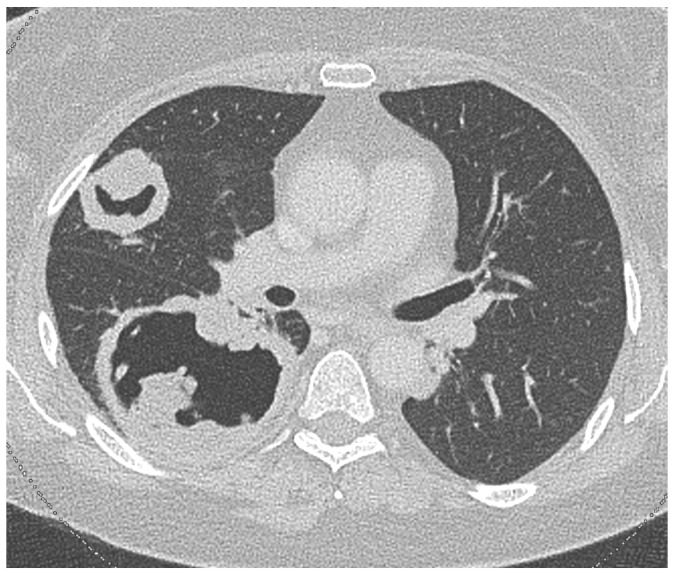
Chest CT: two cavitary lesions in the right upper and lower lobes, with the lower cavity containing a fungal mass with a thickened wall, consistent with aspergilloma. Findings are suggestive of pulmonary aspergillosis. Representative image from the anonymized institutional radiology archive of University Hospital Frankfurt.
